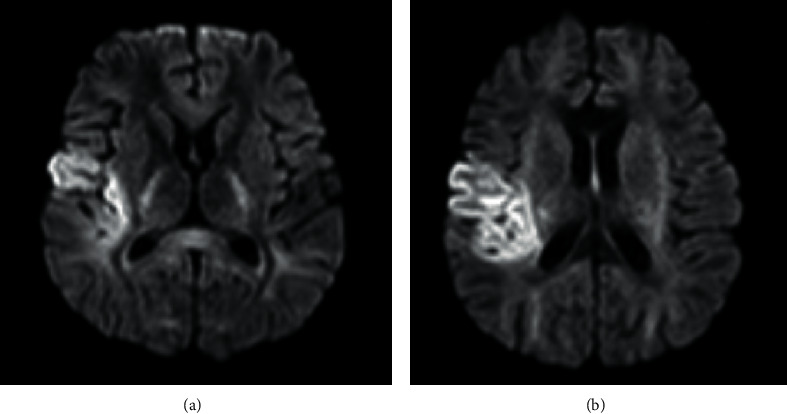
DWI: hyperintensity over right temporal lobe and insular region.
